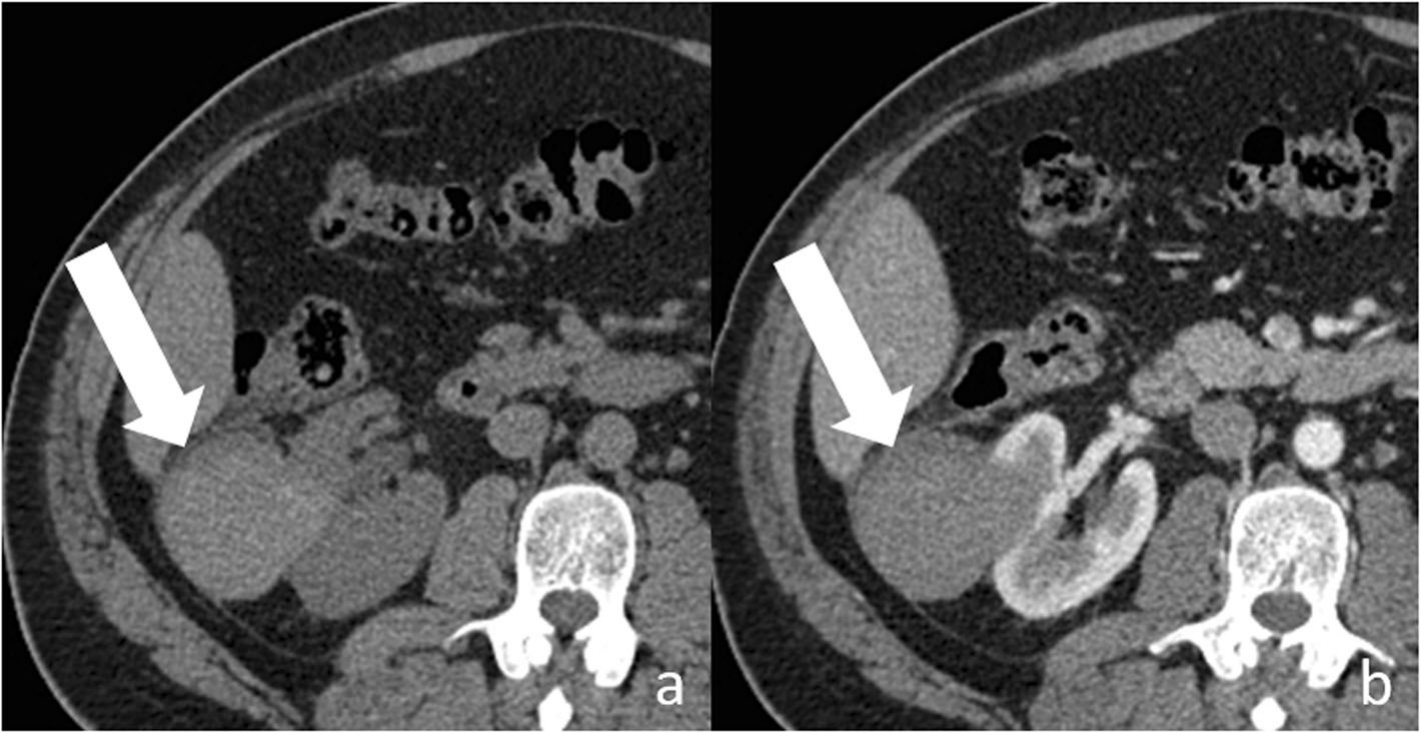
Bosniak category IIF renal cyst. Axial non-enhanced (a) and contrast-enhanced (b) CT images shows a large (> 3 cm) lesion (arrow) with spontaneous hyperattenuation and no enhancement
